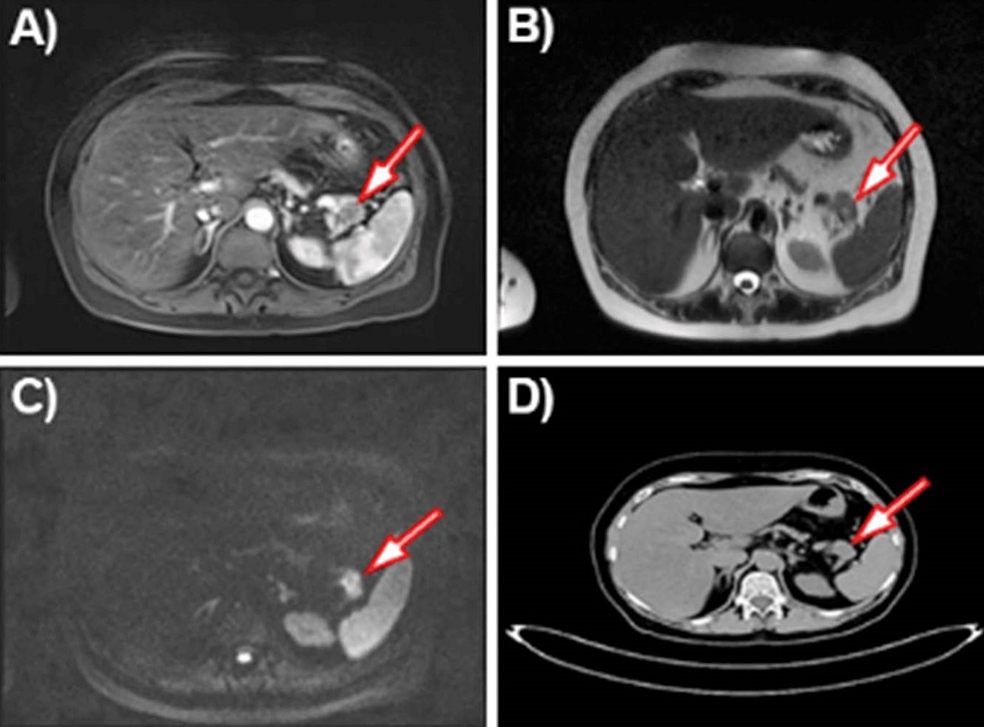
(A) MRI of the abdomen, T1 +contrast; (B) MRI of the abdomen T2; (C) MR DWI (diffusion); (D) Abdominal computed tomography showing tumor mass in the tail of the pancreas. MRI: magnetic resonance imaging, DWI: diffusion-weighted imaging
